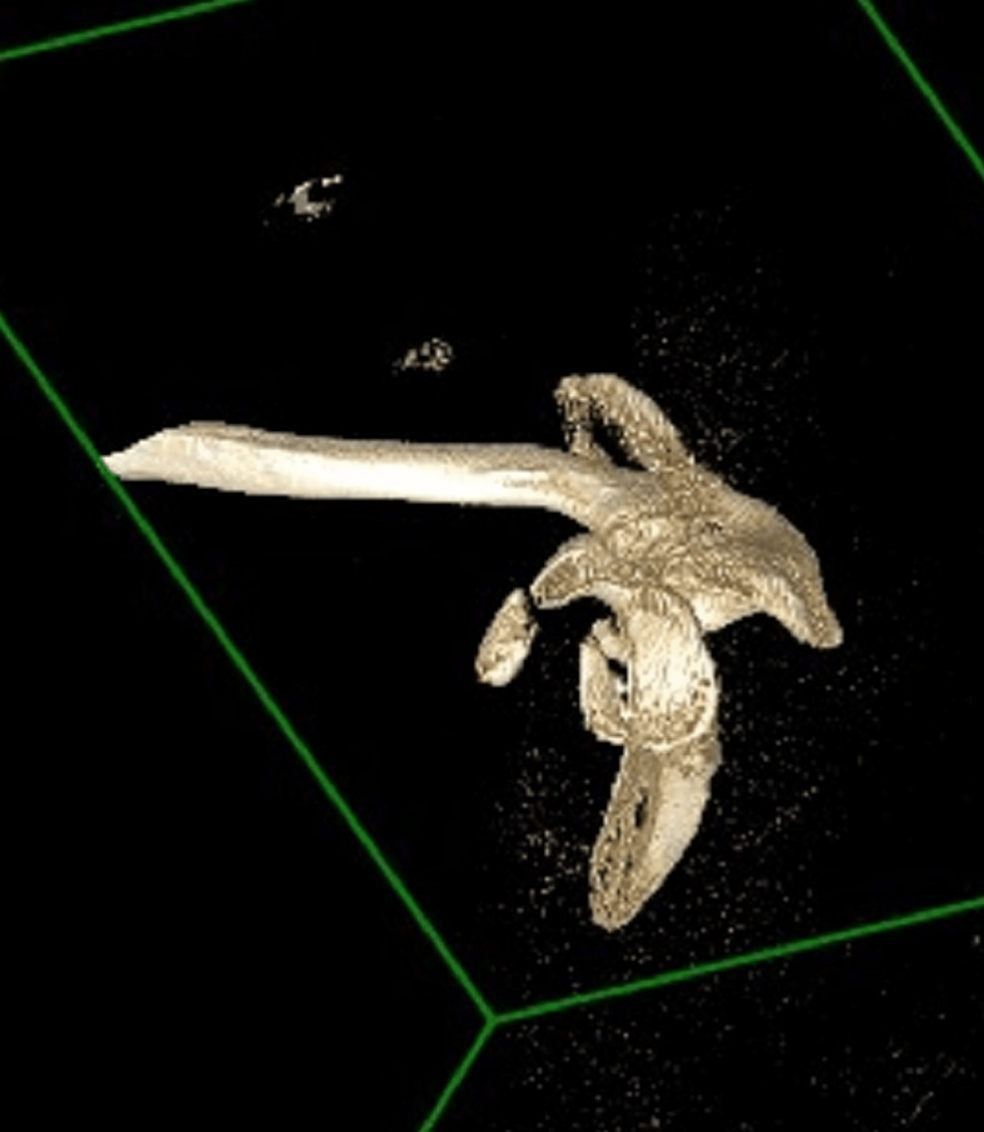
CT reconstruction of the glenoid and coracoid fractures This demonstrates the sizeable anterior glenoid fragment and its comminution, as well as the large coracoid process fragment, which had been displaced.
